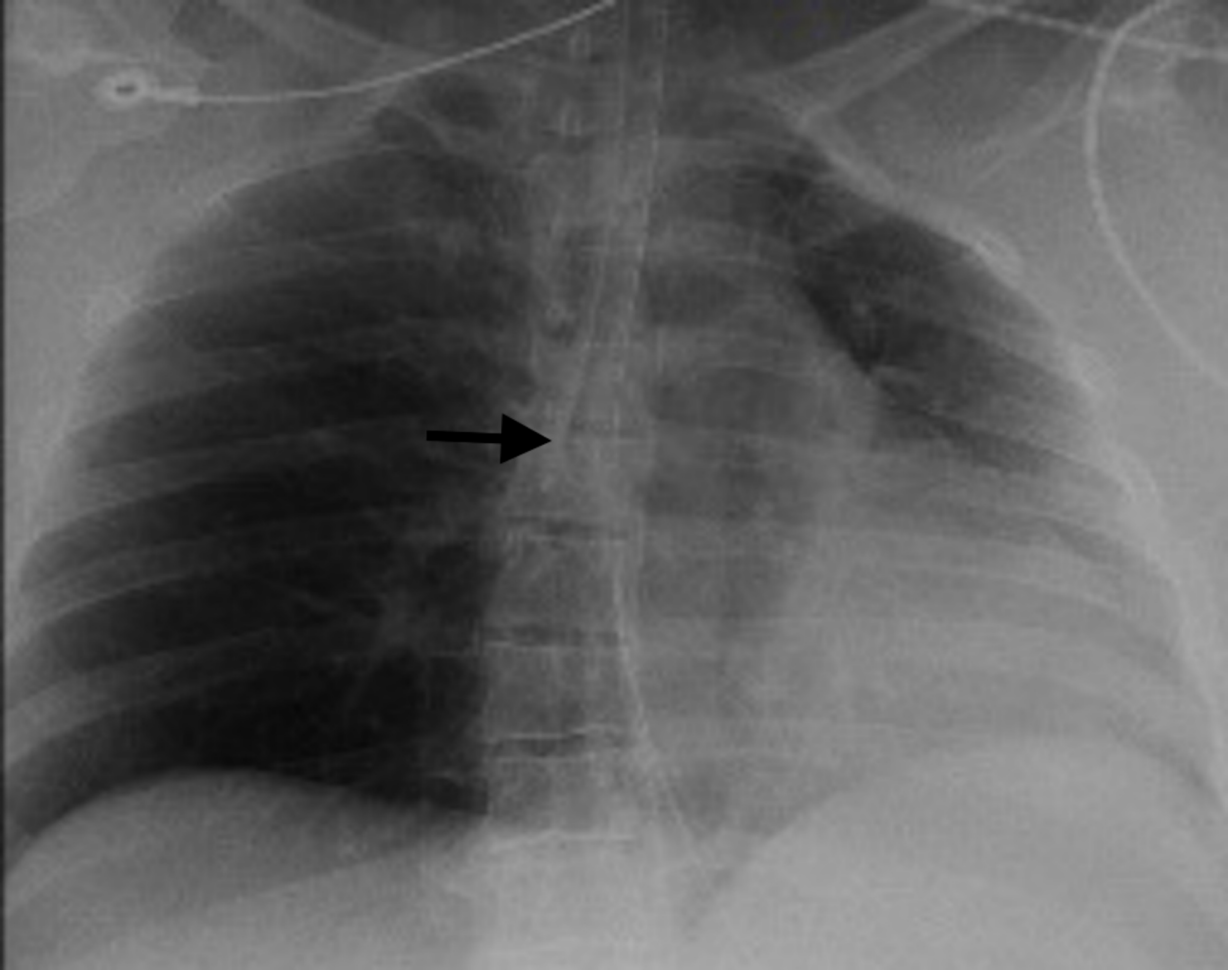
Chest X-ray showing endotracheal tube terminating at the level of carina directed towards the right main stem bronchus (black arrow)
